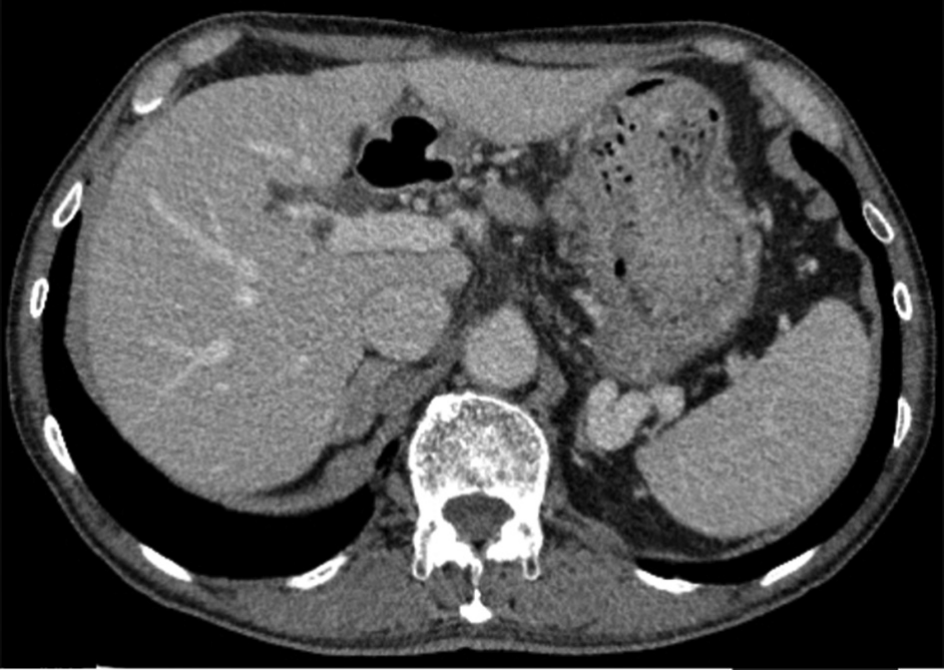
Posttreatment enhanced abdominal CT on postoperative day 34 showed that the abscess had completely resolved.
